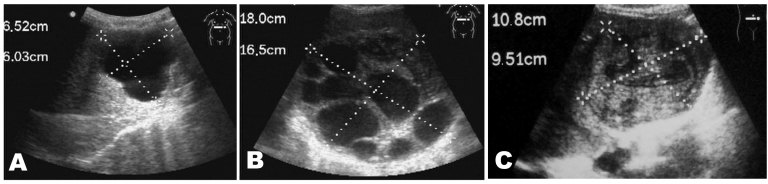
Ultrasonographic images of cystic echinococcosis in the liver in patients from the Yanahuanca district, Central Peruvian Highlands. A) Cyst type CE1; B) Cyst type CE2; C) Cyst type CE4.
